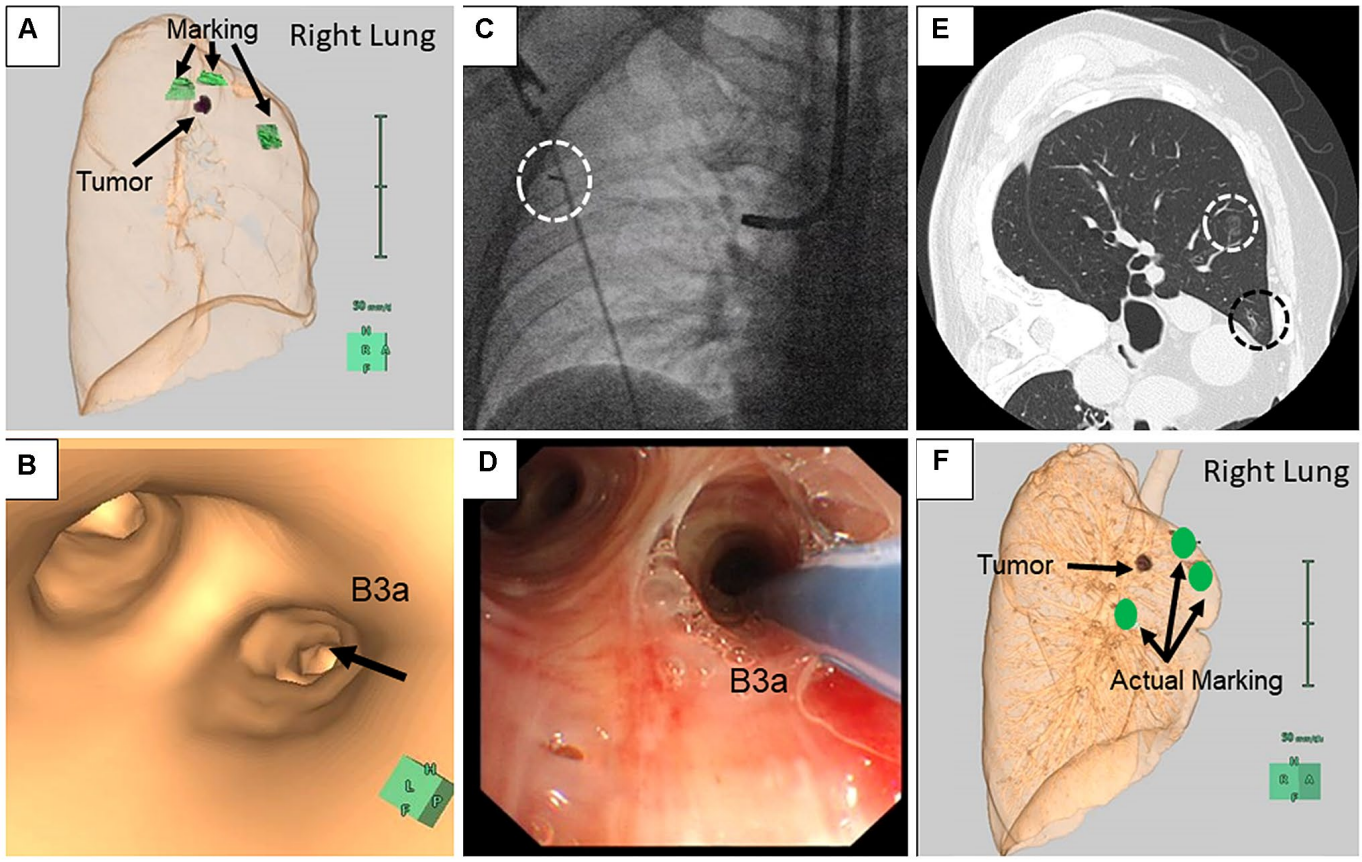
Steps involved in the ICG VAL-MAP procedure. a, b Before the bronchoscopic procedure, lung mapping was planned and a virtual bronchoscopic navigation image was created using Synapse Vincent. c X-ray fluoroscopy confirmed the tip of the catheter (surrounded by a white-dotted circle) reached the pleura. d ICG and IC dyes were injected into the targeted bronchus. e The post-VAL-MAP chest CT image shows the location of the lesion (surrounded by a white-dotted circle) and the actual marking (surrounded by a black-dotted circle). f Three-dimensional image of post-VAL-MAP chest CT was reconstructed for surgery
